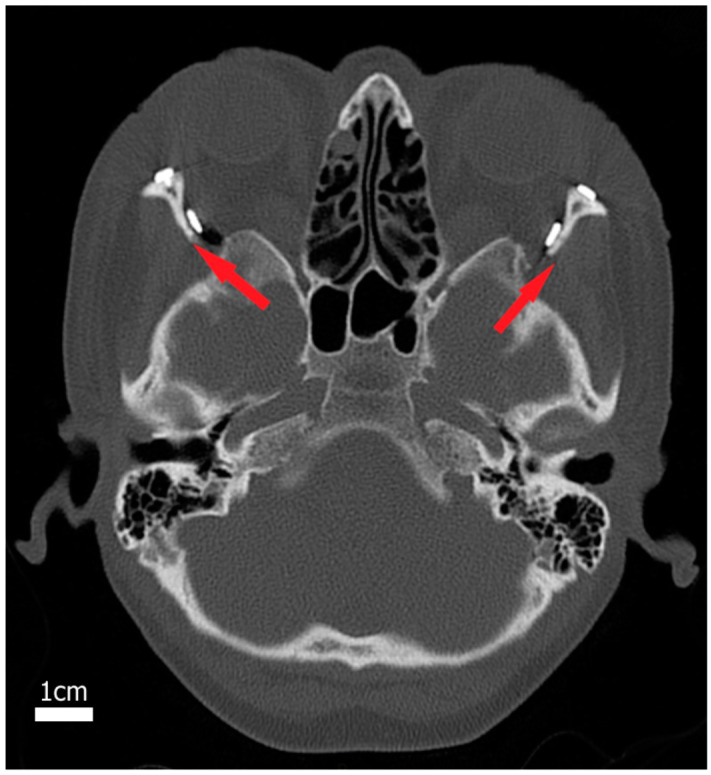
Example of LAVA treatment. A central CT slice of a TED patient who underwent a LAVA treatment. Valgization of the lateral wall at both sites prevents herniation of the temporalis muscle (red arrows) and keeps the new lateral orbital space open.
